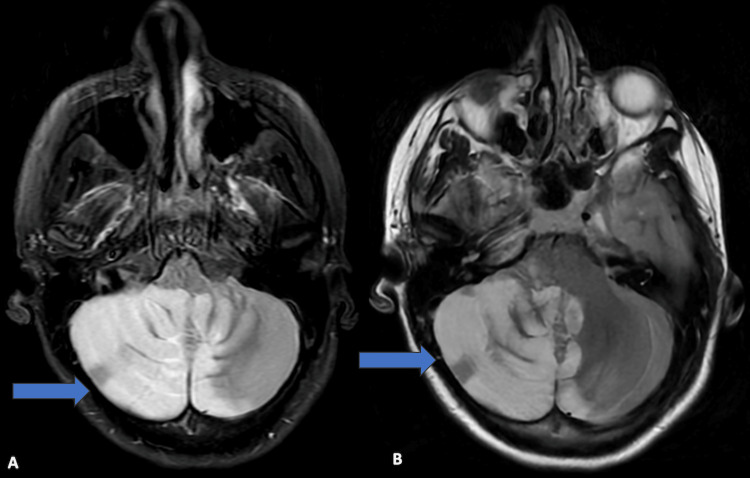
Axial fluid-attenuated inversion recovery (FLAIR) (A) and T2-weighted (B) images showing extensive cerebellar hyperintensity consistent with edema, with effacement of the cerebellar sulci and mass effect on the fourth ventricle.
